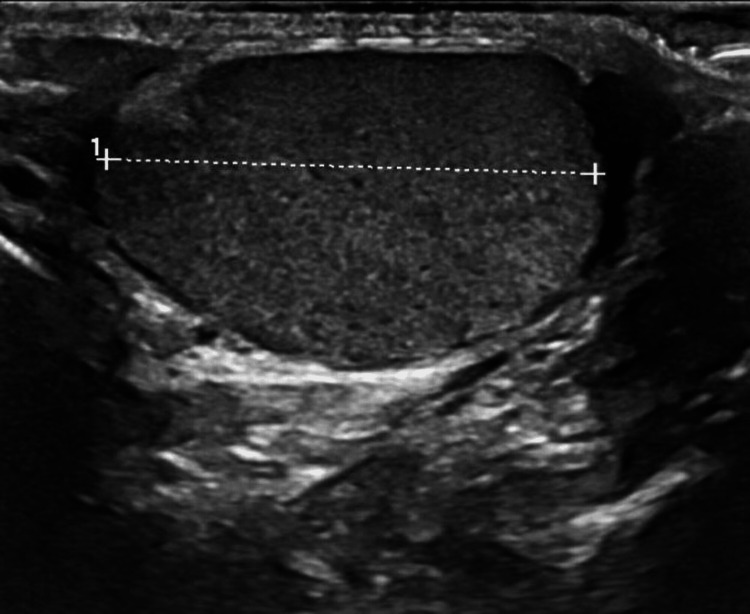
Scrotal ultrasonography revealed an intrascrotal extratesticular mass measuring approximately 3 cm.
